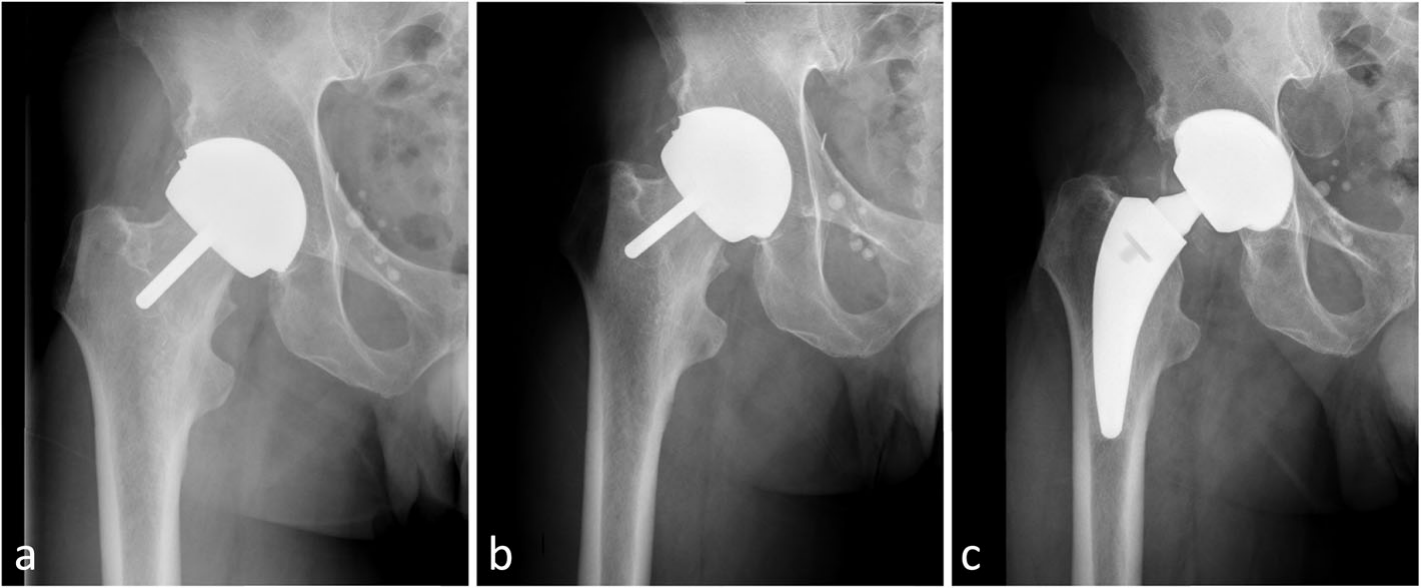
a A 54-year-old man with osteoarthritis underwent implantation of hip resurfacing implant. An AP radiograph of the patient’s right hip taken 4 months after surgery. b An AP radiograph of the patient’s right hip taken 8 years postoperatively shows the femoral component is loose. c The patient underwent bipolar revision with a CoC implant
